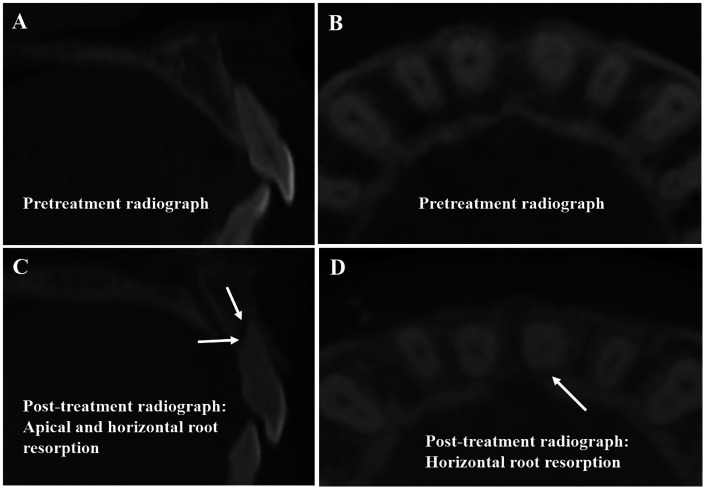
Examples of U1 root resorption: (A,B) pretreatment radiographs. (C,D) Post-treatment radiographs showing apical and horizontal root resorption (arrows).
